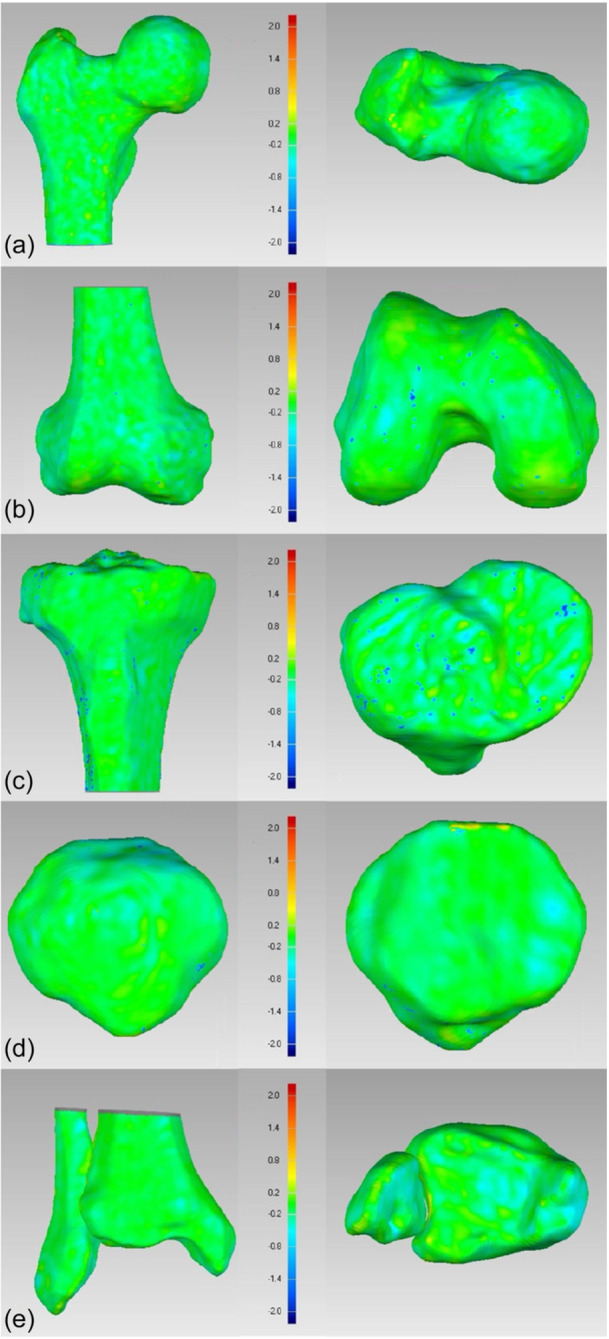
Surface comparison between 3D reconstruction of MSCT and CBCT of the (a) proximal femur (anterior and cranial view), (b) distal femur (anterior and caudal view), (c) proximal tibia (anterior and cranial view), (d) patella (anterior and posterior view), and (e) distal tibia and fibula (anterior and caudal view). The colouring describes the degree of deviation. The scale is presented in mm. CBCT, cone bean computed tomography; MSCT, multislice computed tomography.
